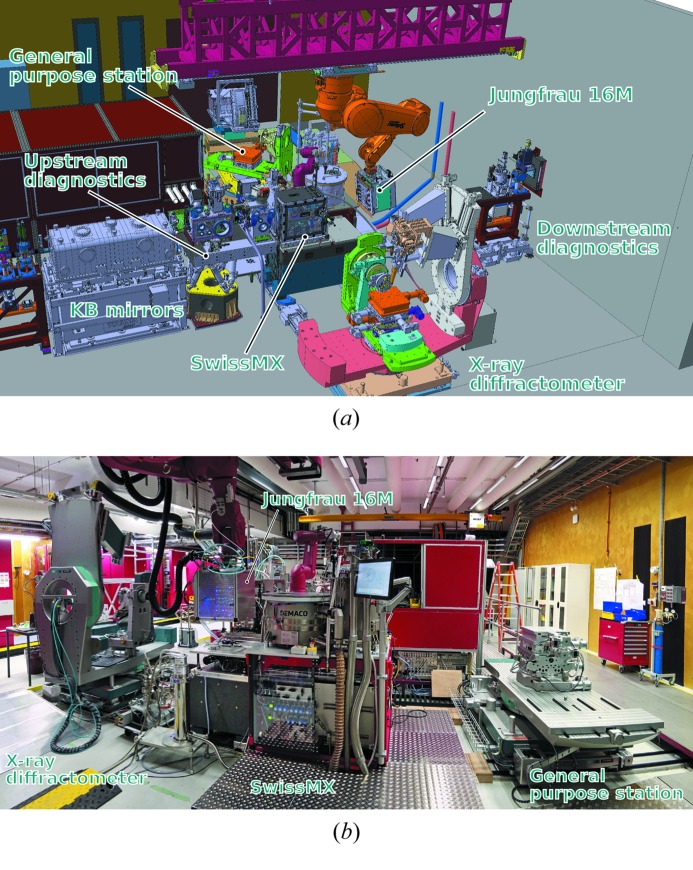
Experimental area and endstations. Top: CAD-view showing the robot detector mount and the three experimental endstations. Bottom: photograph in the direction upstream of X-ray propagation.
